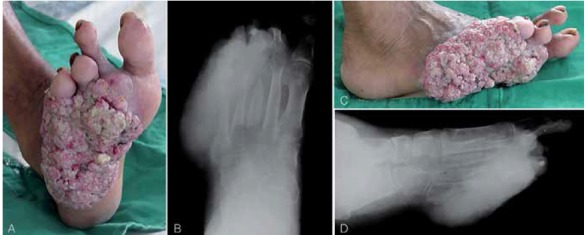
Case 9. Squamous cell carcinoma, ulcerative type; (A) plantar surface of the right foot, (B) anteroposterior X-ray view, (C) lateral surface of the right foot, and (D) profile X-ray view. Note the severe bone destruction caused by tumoral invasion.
